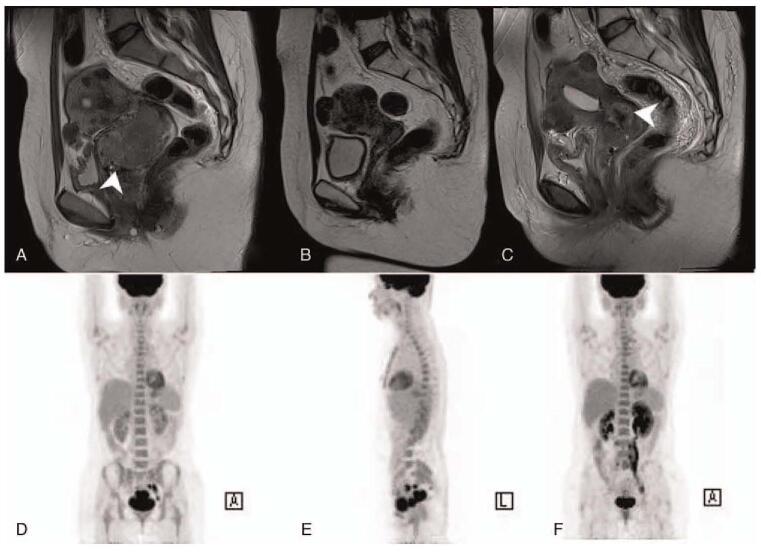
MRI T2 weighted sagittal image shows about 6.8 cm sized mass in cervical canal, induration extended to right parametrium (arrow) (A). Much regression of known cervical cancer after treatment (B). Small nodular lesions in the endocervical canal (arrow) (C). PET-CT show hypermetabolic mass, r/o cervical cancer (max SUV 10.6) and hypermetabolic nodule, in Lt. obturator, Lt. external iliac, r/o metastatic LN (D, E). Hypermetabolic cervical cancer with progression of metastatic LN (F).
